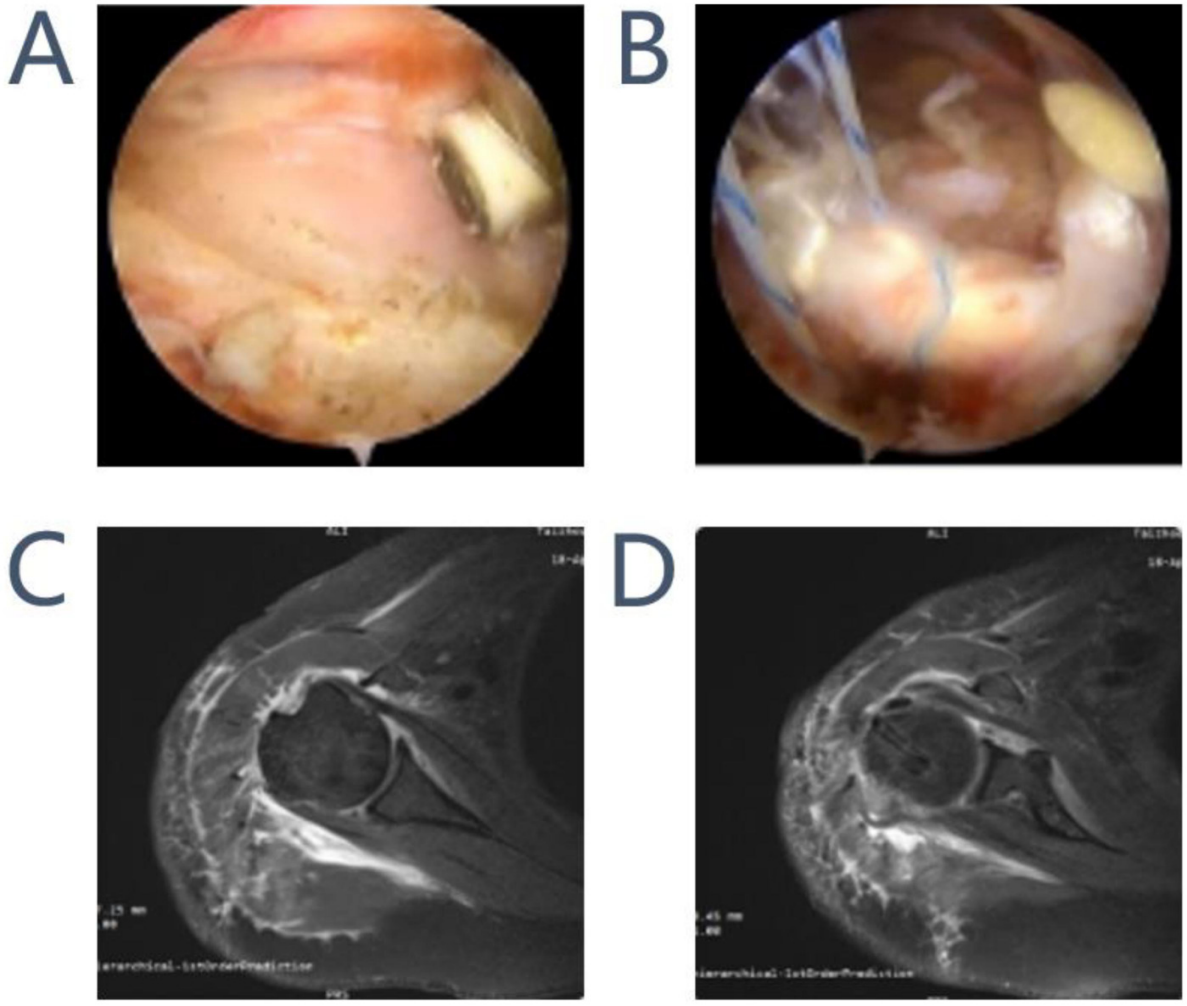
Intraoperative transection of the long head of the biceps brachii tendon (A) and fixation of the long head of the biceps brachii tendon (at the intertubercular groove level) (B) postoperative MRI images of the shoulder joint following transection of the long head of the biceps brachii tendon (C) and the shoulder joint following fixation of the long head of the biceps brachii tendon (D).
